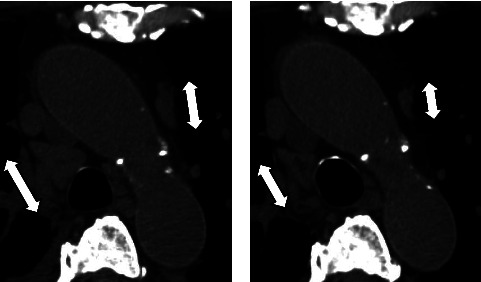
Radiological findings. Computed tomography of the chest before (a) and after treatment (b). Periaortic and lung hilar lymph nodes (two-headed arrow) appear reduced in size.
